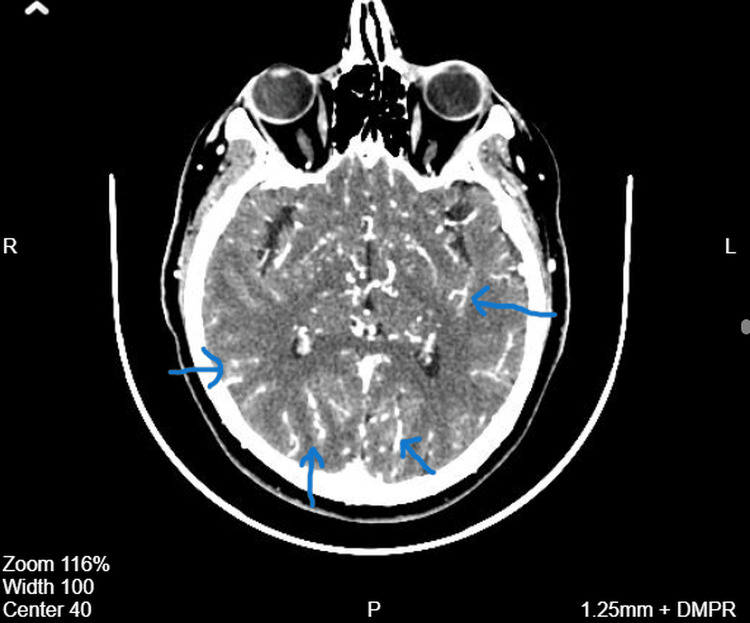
Contrast-enhanced axial CT image demonstrating abnormal collateral vasculature in Moyamoya disease Contrast-enhanced axial computed tomography (CT) image of the brain demonstrating multiple abnormal collateral vessels within the cerebral hemispheres. These tortuous vascular structures represent compensatory collateral circulation characteristic of Moyamoya disease, which develops secondary to progressive stenosis of the distal internal carotid arteries and their proximal branches. Blue arrows indicate the abnormal collateral vessels.
